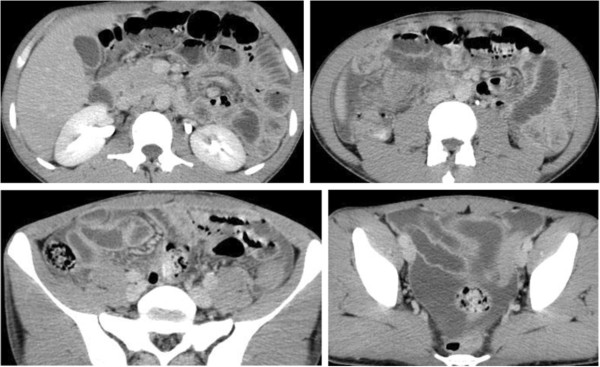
Abdominal CT scan on admission. Ascites and intestinal swelling can be seen.
